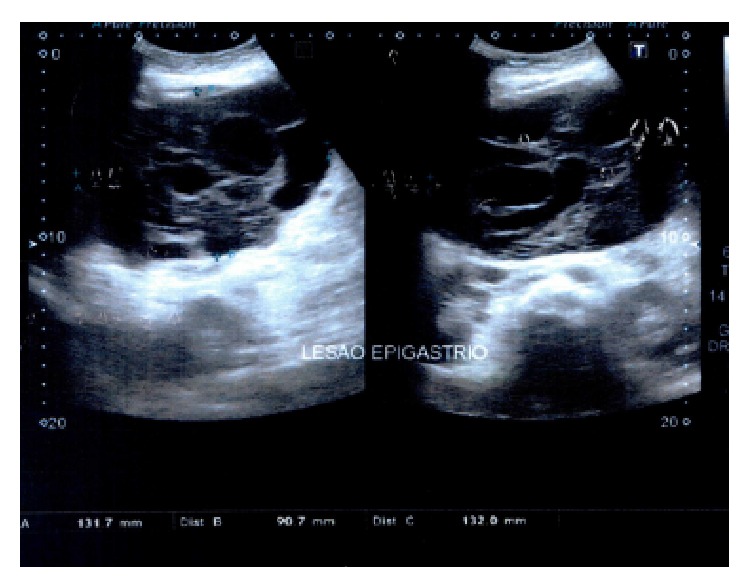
Abdominal ultrasonography showing a huge heterogeneous mass.
